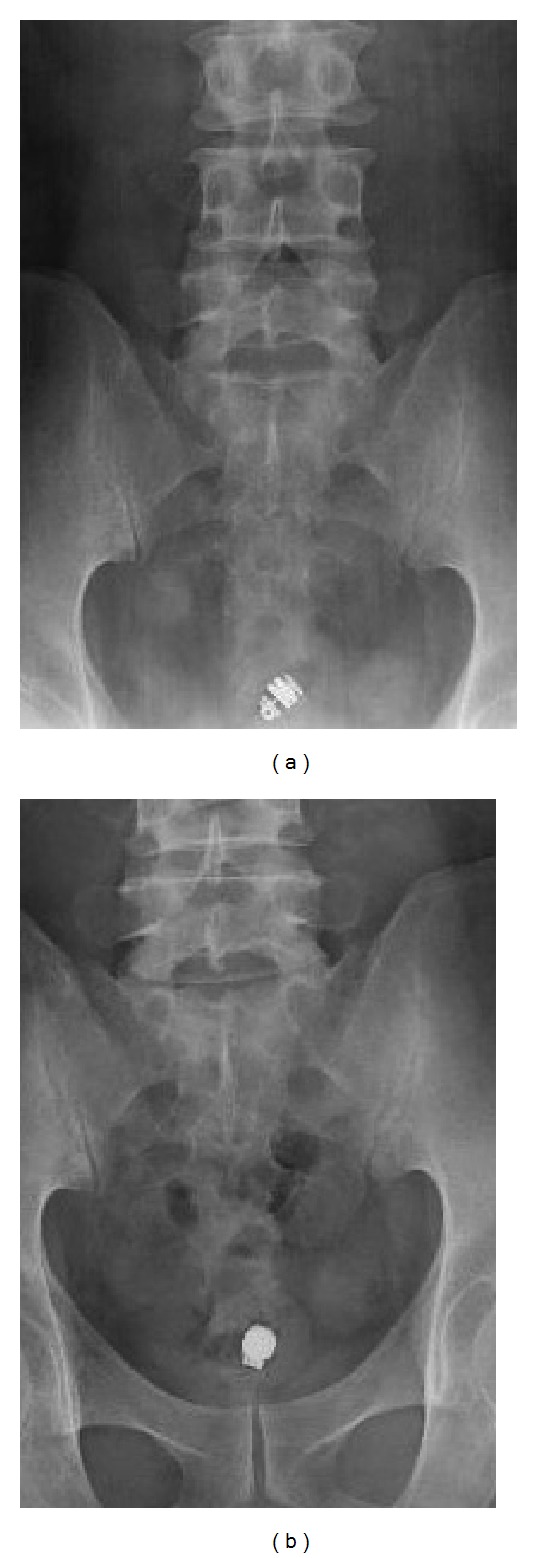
Abdominal X-ray image visualizing a CE capsule in the pelvic cavity. The capsule retention might have been overlooked if the range of the X-ray image had shifted slightly (a). Follow-up X-ray after 7 days revealed that the capsule remained at the same place (b).
